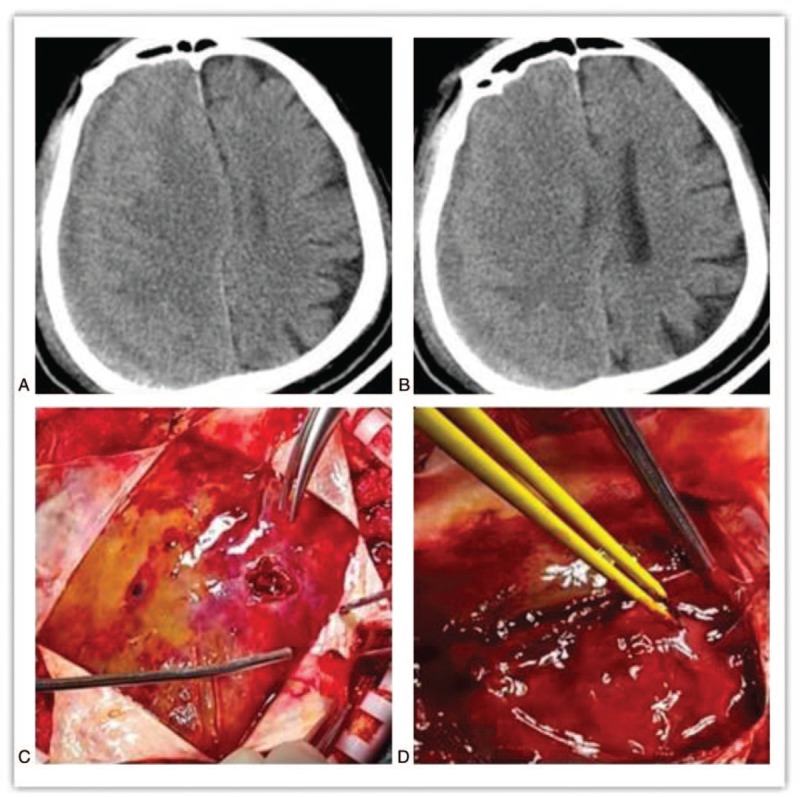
Findings during preoperative CT are shown in A and B: slightly high bands were observed below the right frontotemporal top plates with a low-density shape, compressed right brain tissues, and a shallow cerebral sulcus that then disappeared. The right ventricle was compressed and decreased in size. The median line was shifted toward the left side. Intraoperative images are shown in C and D: A large amount of yellow-white purulent fluid and bloody fluid were found subdurally of the frontotemporal region, which showed a complete capsule and obvious local separation.
